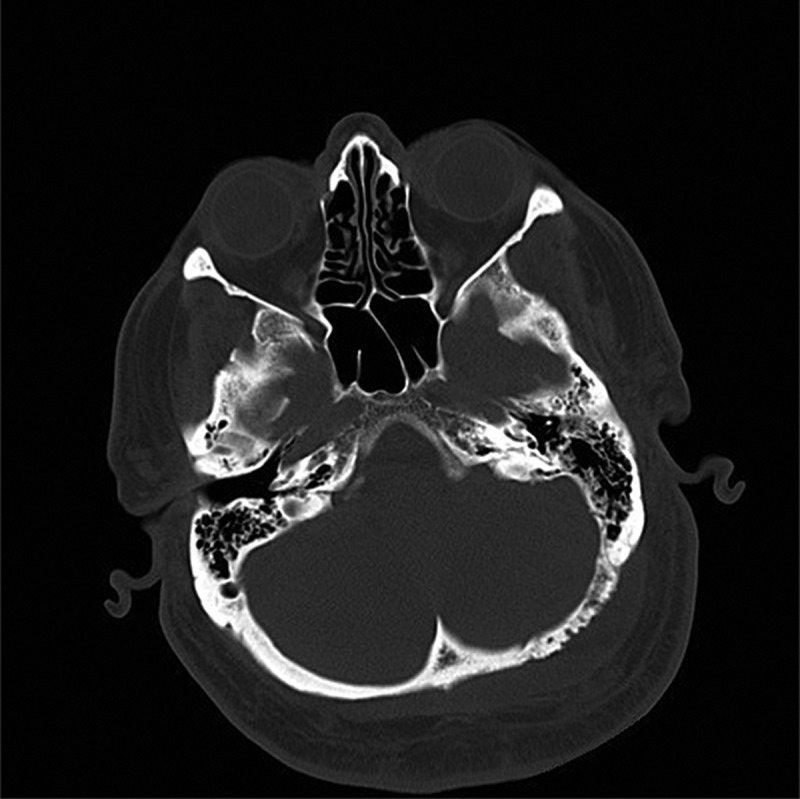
CT scan showing mottled appearance of left occipital bone, consistent with osteomyelitis.
